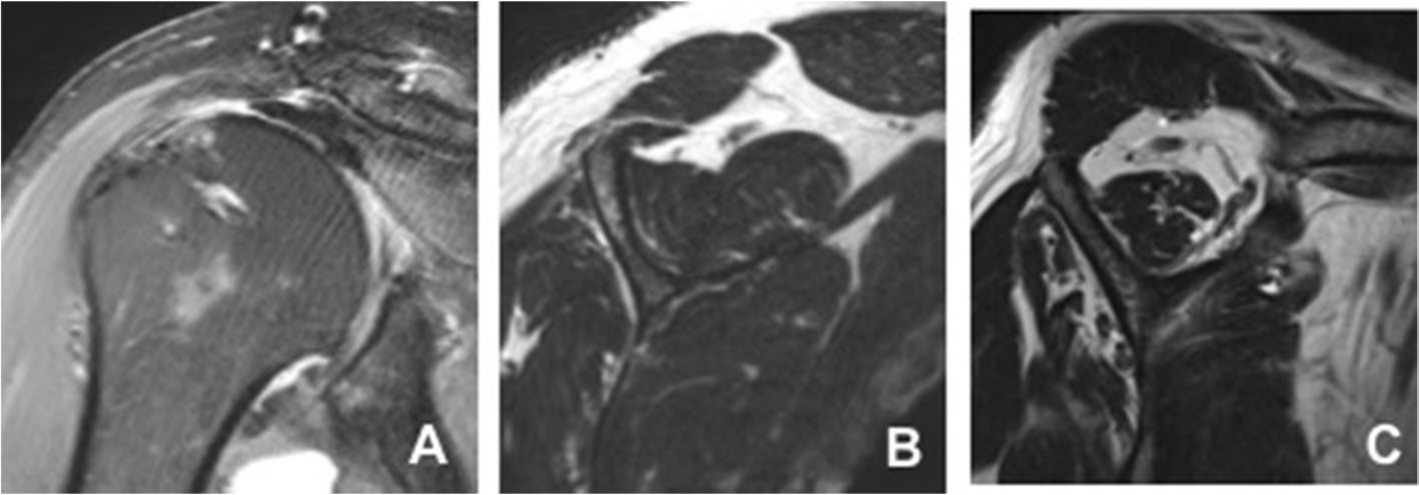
A Postoperative coronal MRI showing a supraspintatus tendon re-tear at final follow up (Sugaya Type IV). B Preoperative parasagittal MRI of supraspinatus muscle showing slight atrophy. C Postoperative parasagittal MRI of supraspinatus muscle showing progression of atrophy and fatty infiltration due to RC retear
